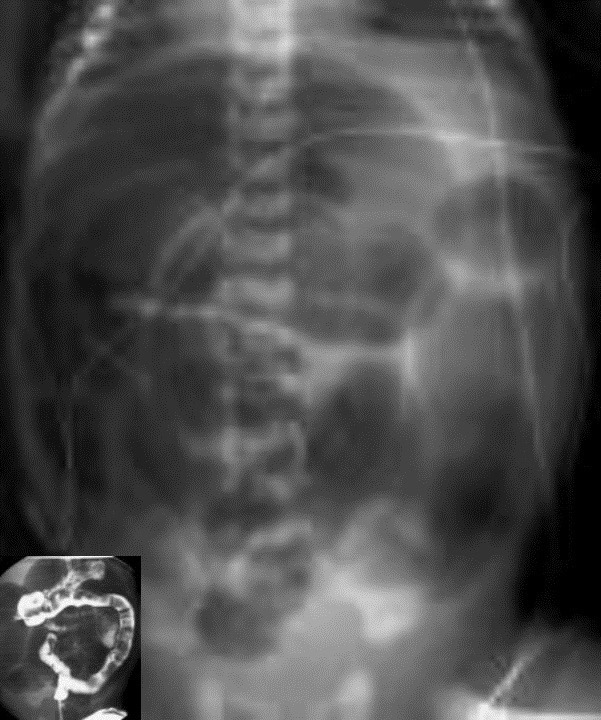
Figure 12: X-ray showing dilated bowel loops. Contrast enema showed filling defects seen in meconium plug syndrome.
